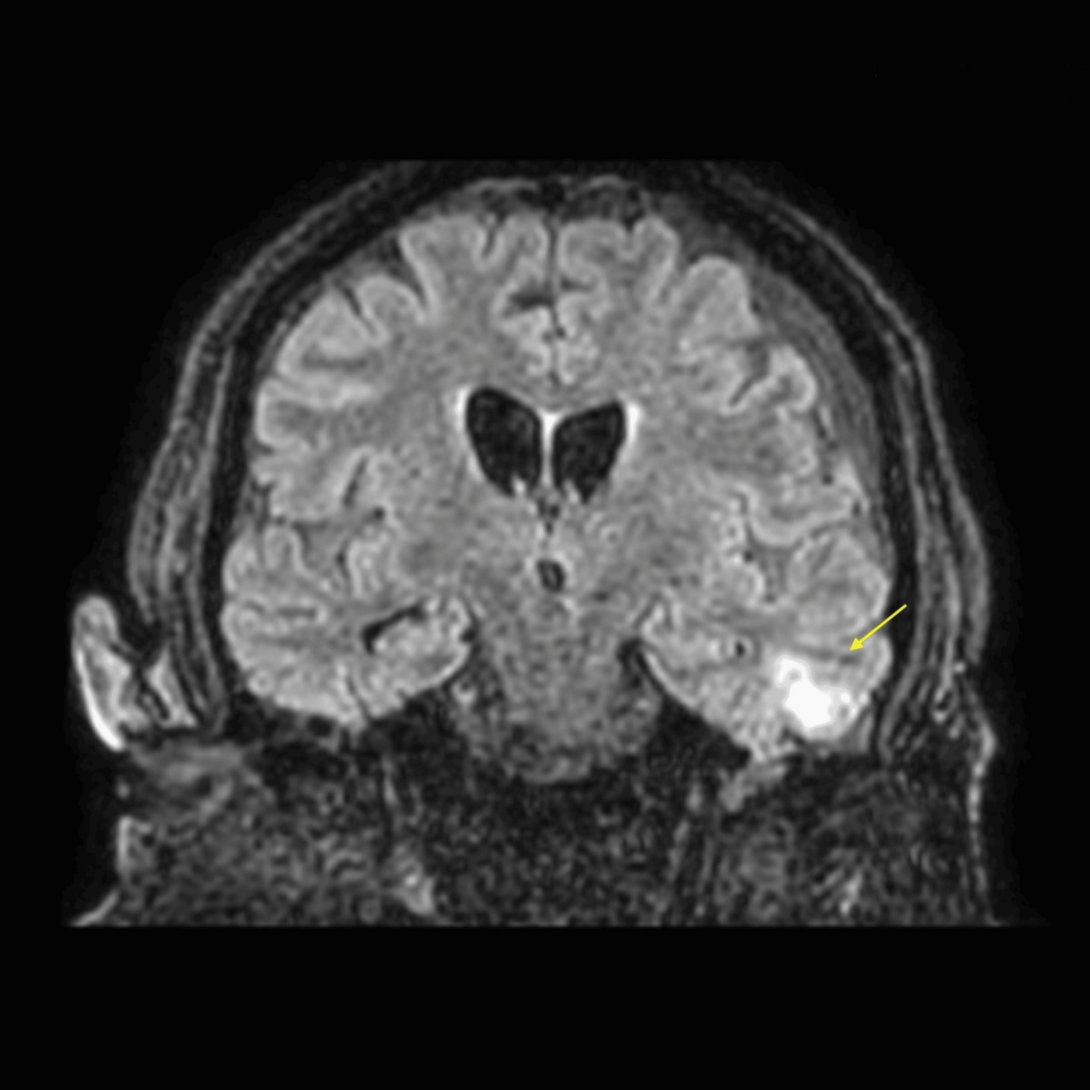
Brain magnetic resonance imaging Magnetic resonance imaging showing a left frontotemporal subdural collection and a left temporobasal abscess with mild surrounding edema (yellow arrow), associated with bilateral otomastoiditis and left-sided petrous bone erosion, consistent with otogenic intracranial spread.
